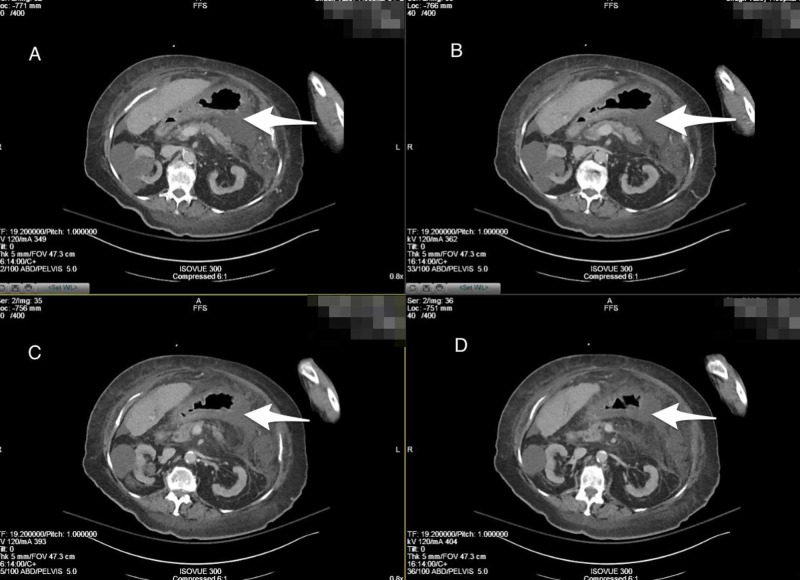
CT Abdomen (axial plane) A-D) Cross-sectional view of abdomen showing fluid around the gastric area.
